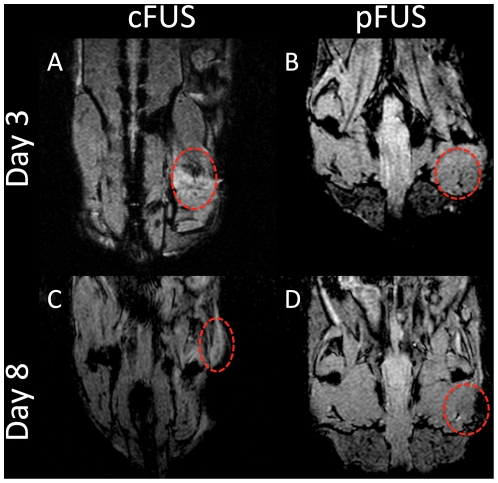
Evaluation of FL-SPION-labeled macrophage migration to FUS-treated muscle tissue by MRI. *T*
